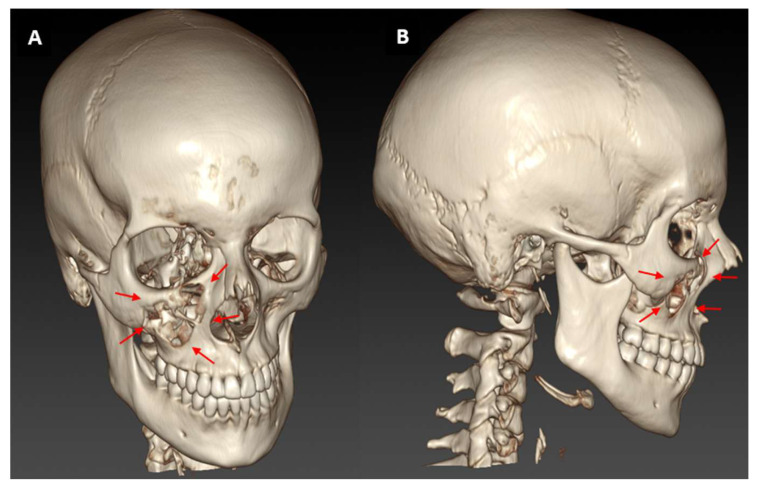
Maxillary fracture following MMA exposure: Frontal (A) and sagittal (B) views of 3D CT image of a 25-year-old female patient who presented to the Department of Oral and Maxillofacial Surgery after being impacted on the right zygomatic bone by an elbow during martial arts. The patient reported persistent dizziness, blurred vision in the left eye and headache, showing a fracture of the anterior wall of the right maxillary sinus with impaction of several lamellar bone fragments into the maxillary sinus and a non-displaced fracture of the lateral wall of the right maxillary sinus. In this case, the patient had no malocclusion, and the maxilla was fixed, which led to a conservative approach. Red arrows indicate the maxillary fracture on the right side.
